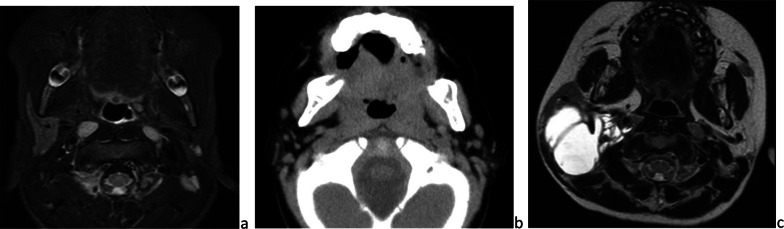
Parotid gland aplasia. Unilateral, isolated aplasia on axial fat sat *T*_2_-weighted image (a). Bilateral aplasia on axial CT image, in Treacher-Collins syndrome (b). Right parotid gland aplasia associated with contralateral macrocystic lymphangioma, on axial *T*_2_-weighted image (c)
